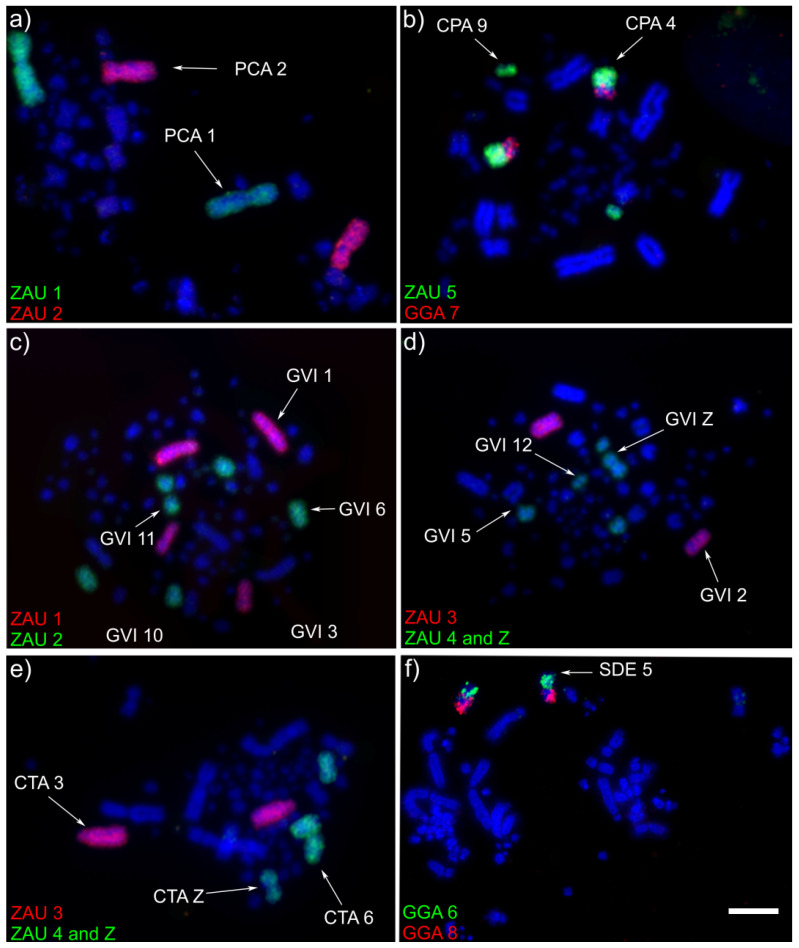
Representative FISH results using different sets of chromosome probes from *Gallus gallus* (GGA) and *Zenaida auriculata* (ZAU) probes on chromosomes of different Columbidae species: (a) *Patagioenas cayennensis* (PCA), (b) *Columbina passerina* (CPA), (c) *Geotrygon violacea* (GVI), (d) *Geotrygon violacea* (GVI), (e) *Columbina talpacoti* (CTA), (f) *Streptopelia decaocto* (SDE). The chromosome probes used are indicated on the left bottom, in green (fluoroscein labeled) or red (biotin-cy3 labeled). Scale bar 10 μm.
